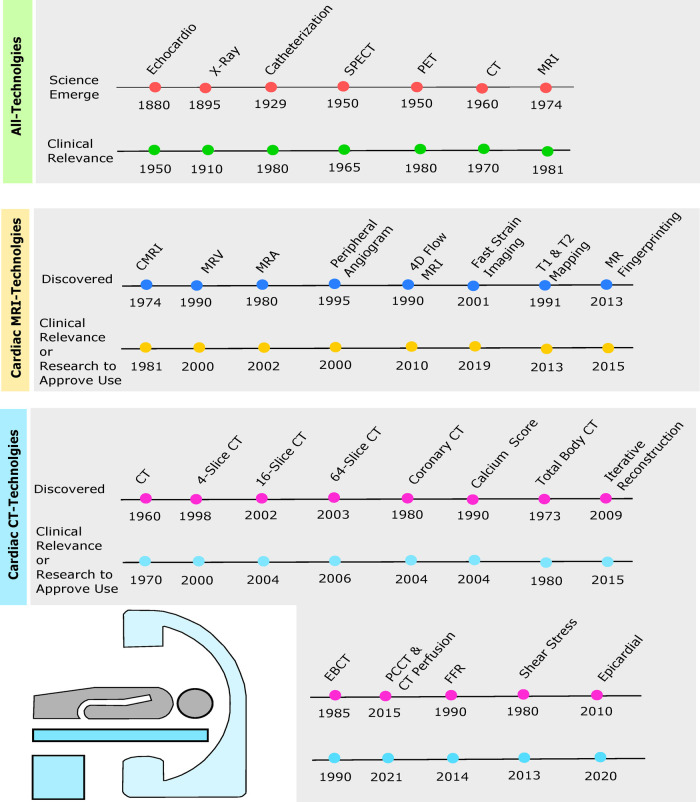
Timelines depict originating year of cardiac imaging invention followed by the year of clinical relevance or enough evidence from research to be approved by the Food and Drug Administration (FDA) for clinical use. Green timeline includes echocardiogram, chest x-ray, cardiac catheterization, SPECT, PET, cardiac CT, and cardiac MRI. Yellow timeline depicts cardiac MRI, magnetic resonance venography (MRV), magnetic resonance angiography (MRA), peripheral MRA, 4D flow, fast strain-encoded (fast-SENC) cardiac imaging, T1 and T2 mapping, and cardiac magnetic resonance (MR) fingerprinting (MRF). Blue timeline shows cardiac CT, 4-slice CT, 16-slice CT, 64-slice CT, coronary CT, calcium score, total body CT, iterative reconstruction (IR), electron beam CT (EBCT), photon-counting CT (PCCT), fractional flow reserve (FFR), wall shear stress (WSS), and epicardial fat enhancement technology.
